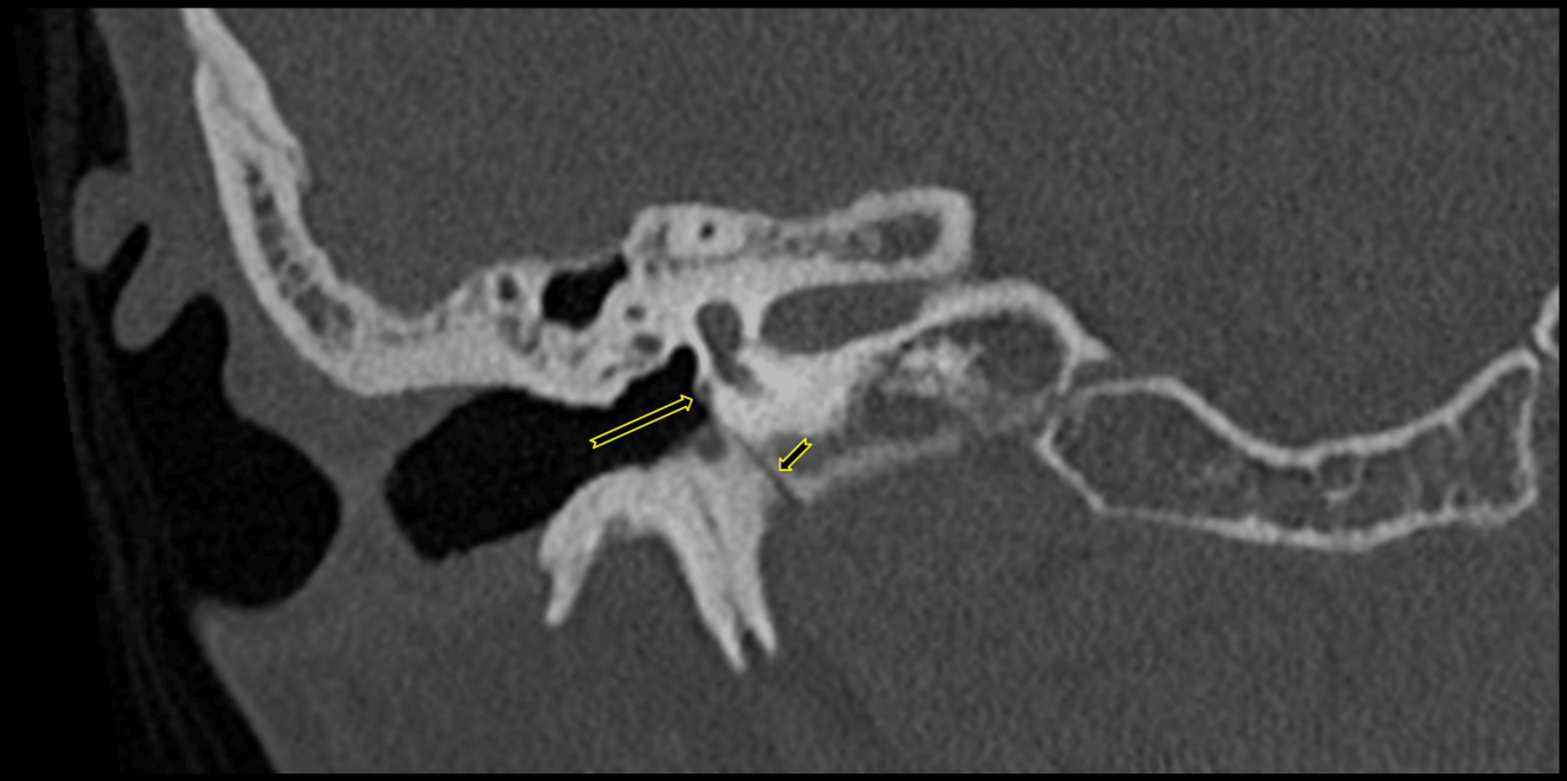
Right temporal bone coronal oblique CT reconstructions demonstrate a small vascular channel of the PSA. A small vascular channel of the PSA (short arrow) leaves the carotid canal, coursing superiorly over the lateral surface of the cochlear promontory (long arrow). PSA: persistent stapedial artery
